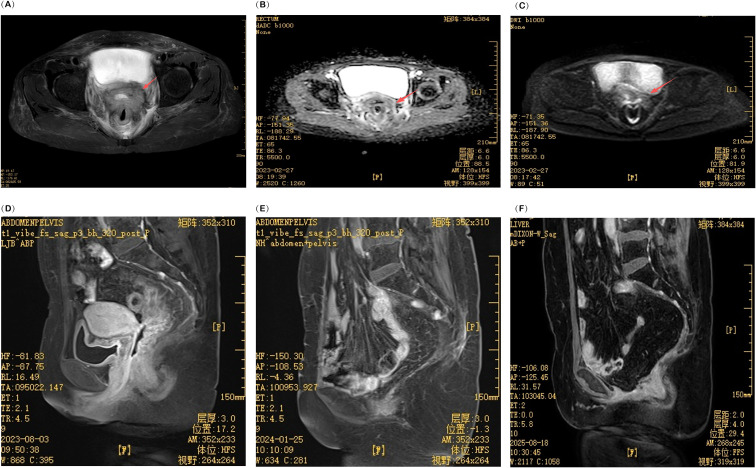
Serial MRI assessment of treatment response and follow-up. (A–C) Pelvic MRI performed on Feb. 27, 2023, after completion of radiotherapy, demonstrating marked tumor regression and substantial reduction of local invasion. (D) Follow-up pelvic MRI obtained on Aug. 3, 2023, showing no radiologic evidence of residual or recurrent disease. (E) Pelvic MRI on Jan. 5, 2024, confirming sustained complete response without signs of local recurrence. (F) Pelvic MRI on Aug. 18, 2025, demonstrating continued absence of tumor recurrence during long-term follow-up.
